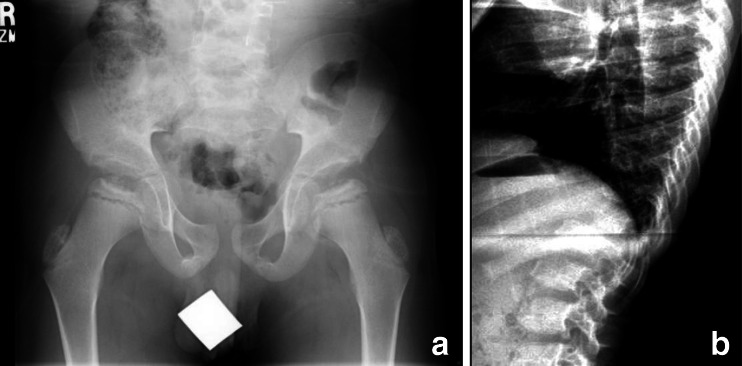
Radiographs of patient 5, male, MPS IVA. a AP pelvis and hips, age 7 years: dysplastic superiorly eroded CFE. b Lateral spine, age 9 years: kyphosis; mildly hypoplastic T11 and T12 and posterior scalloping
